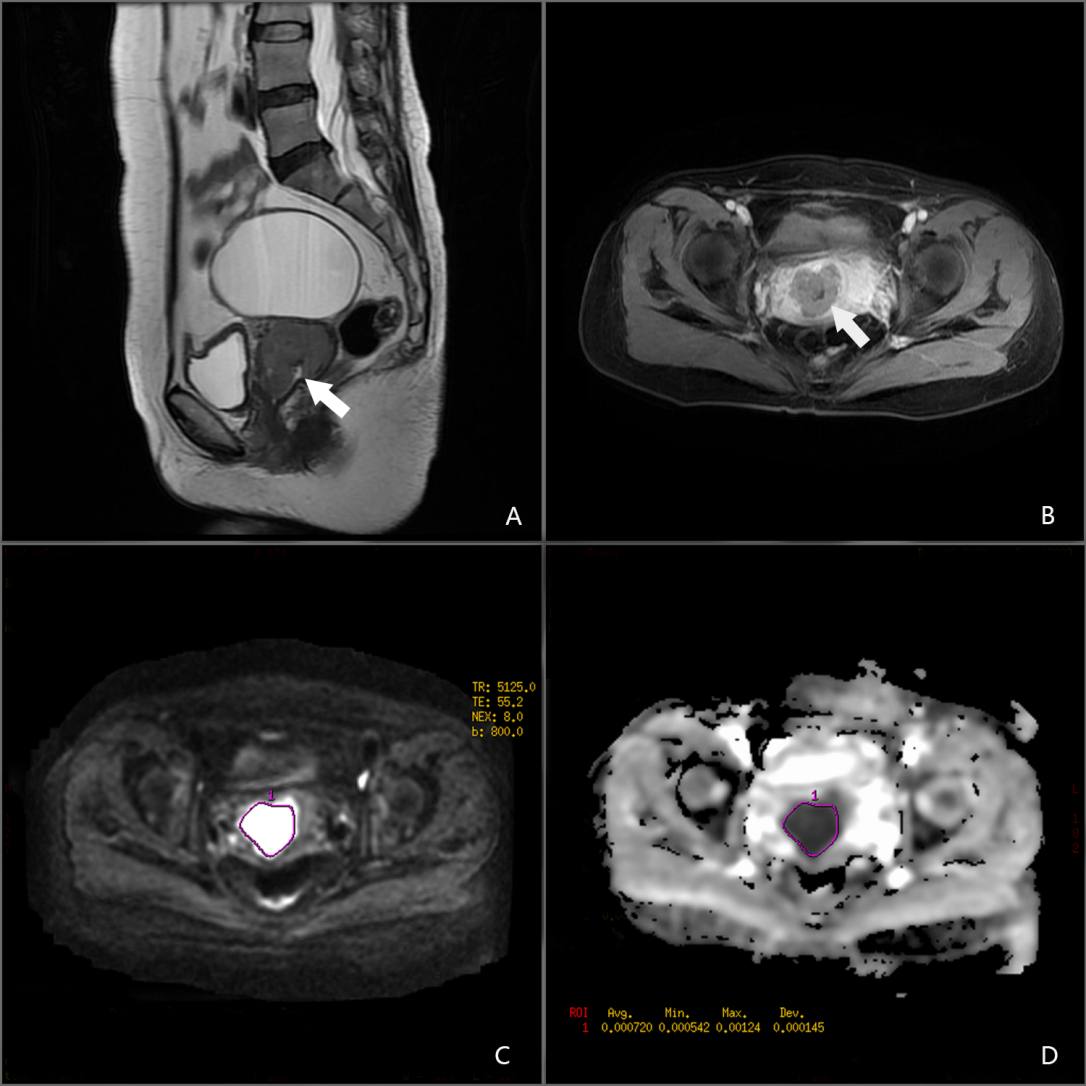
A 45-year-old female patient with NECC. (A) Sagittal T2-weighted image. (B) Axial T1-enhanced image. (C) DWI in *b* = 800. (D) ADC map.
